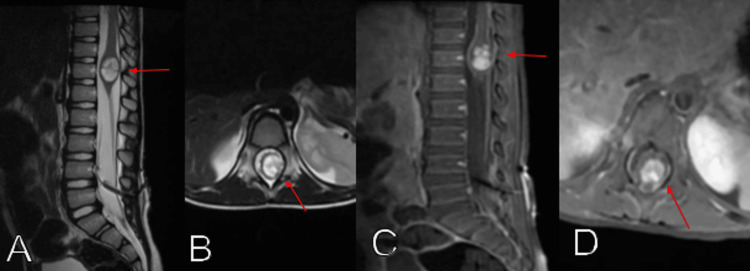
Spine MRI T2-weighted image, sagittal (A) and axial (B) views, and T1-weighted image with gadolinium, sagittal (C) and axial (D) views, showing an oval-shaped intramedullary mass (red arrows) at the T12 level causing expansion of the conus medullaris.
